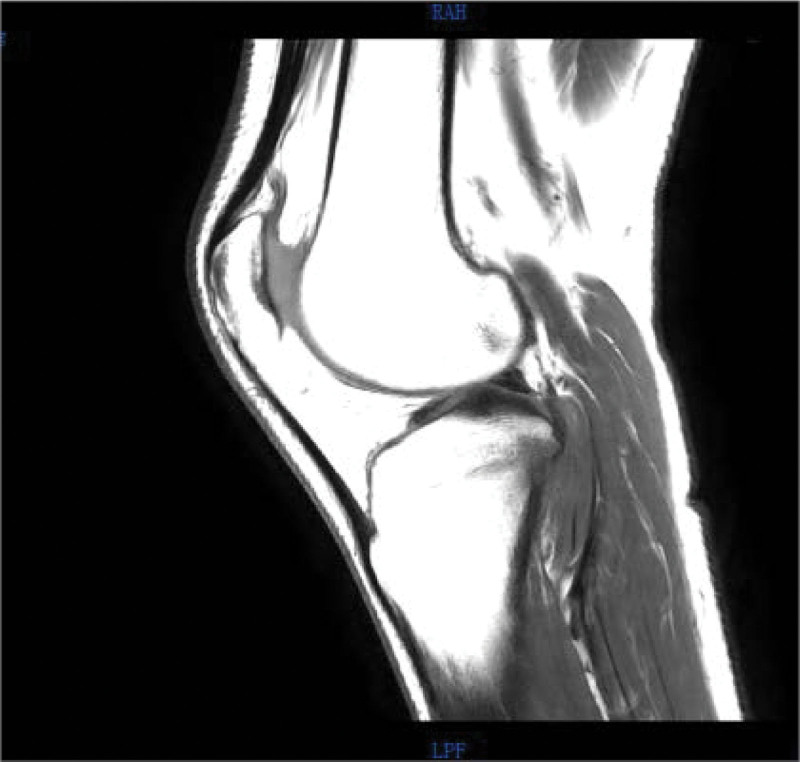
Preoperative MRI T1 sequence in sagittal plane. MRI = magnetic resonance imaging.
